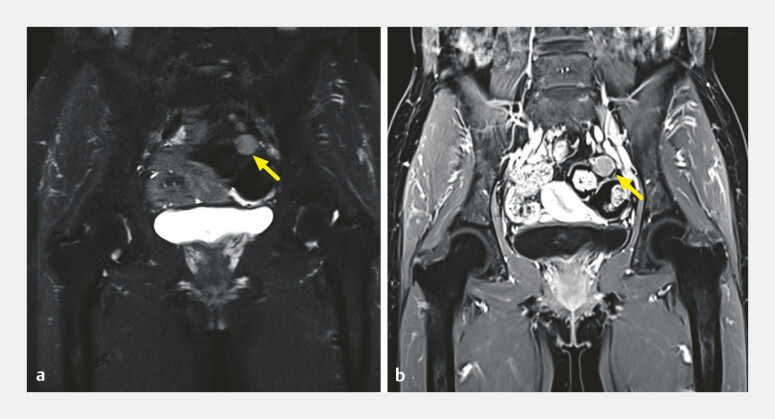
Abdominal magnetic resonance imaging (MRI).
